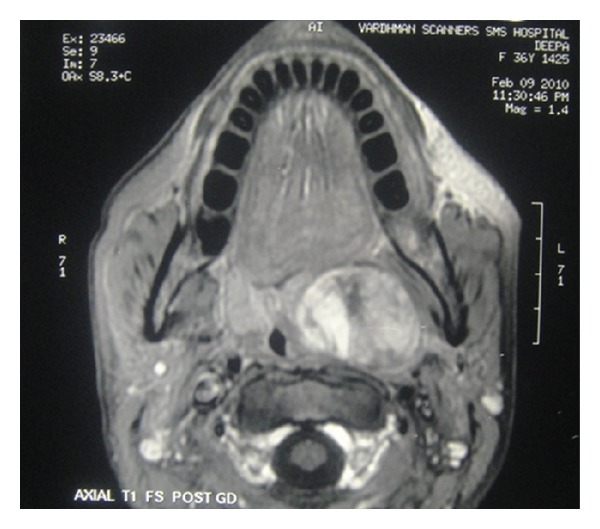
T1-weighted post-Gd MRI large showing heterogenous mass in left parapharyngeal space.
